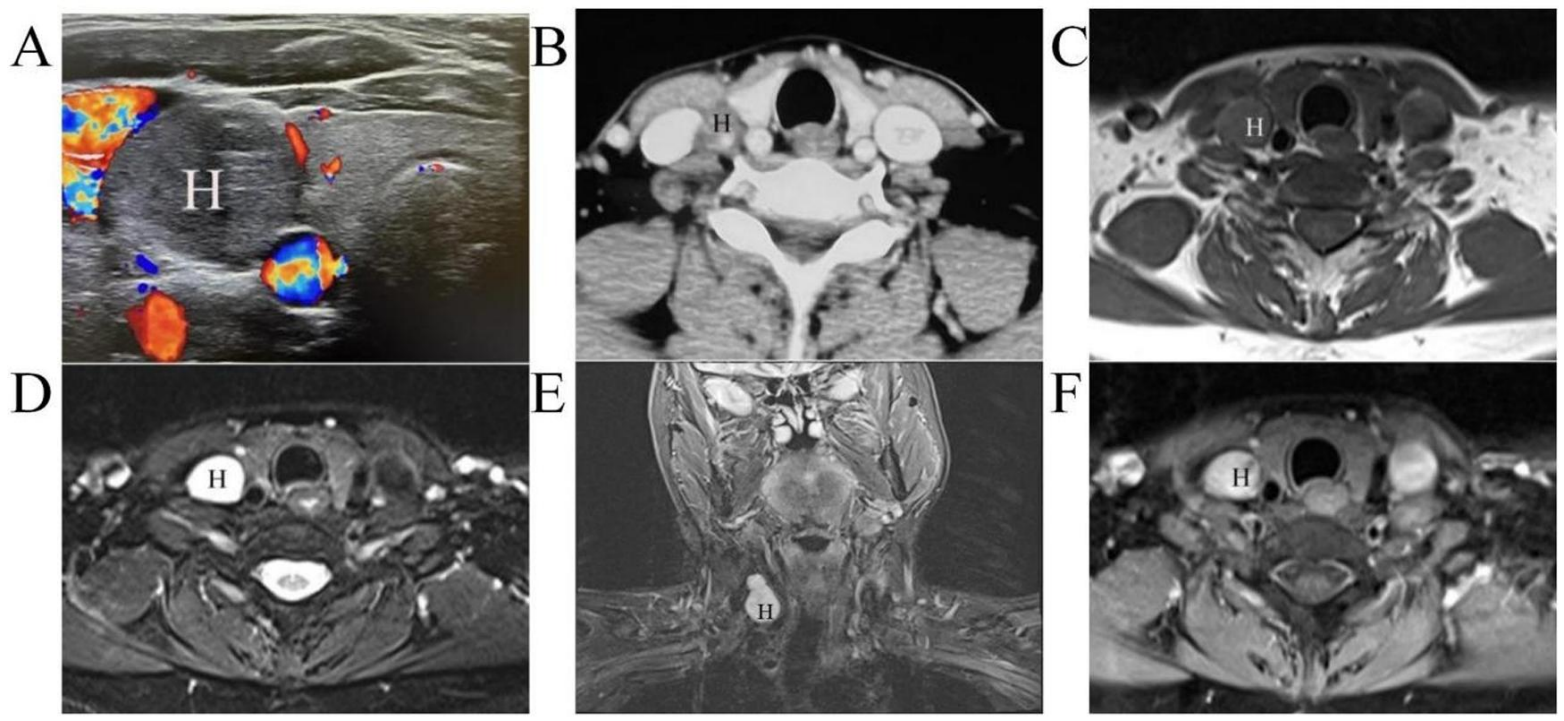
Preoperative imaging of the neck lesion. Ultrasonography of axial section (A) Enhanced computed tomography of axial section (B) T1-weighted imaging of axial section (C); T2-weighted imaging with DIXON fat suppression of axial section (D), and TIRM fat suppression of coronal section (E); enhanced T1-weighted imaging with DIXON fat suppression of axial section (F) H, cavernous hemangioma.
